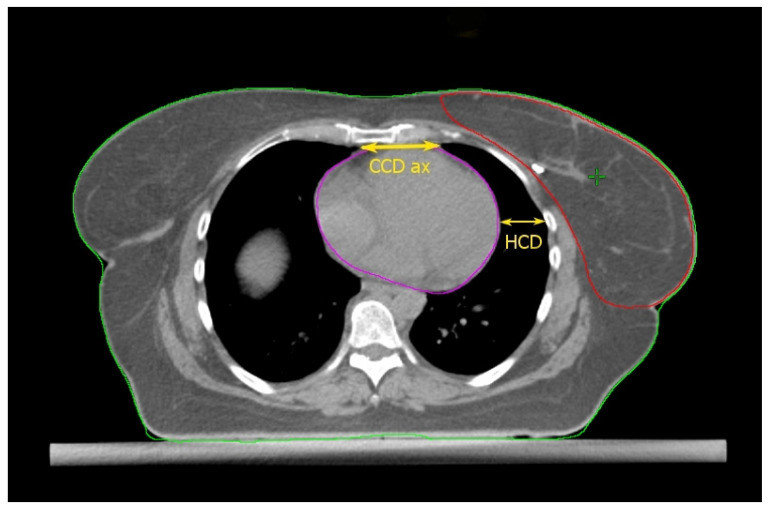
Anatomical parameters on the axial CT plane passing through the liver dome. Read the text for the explanation.
